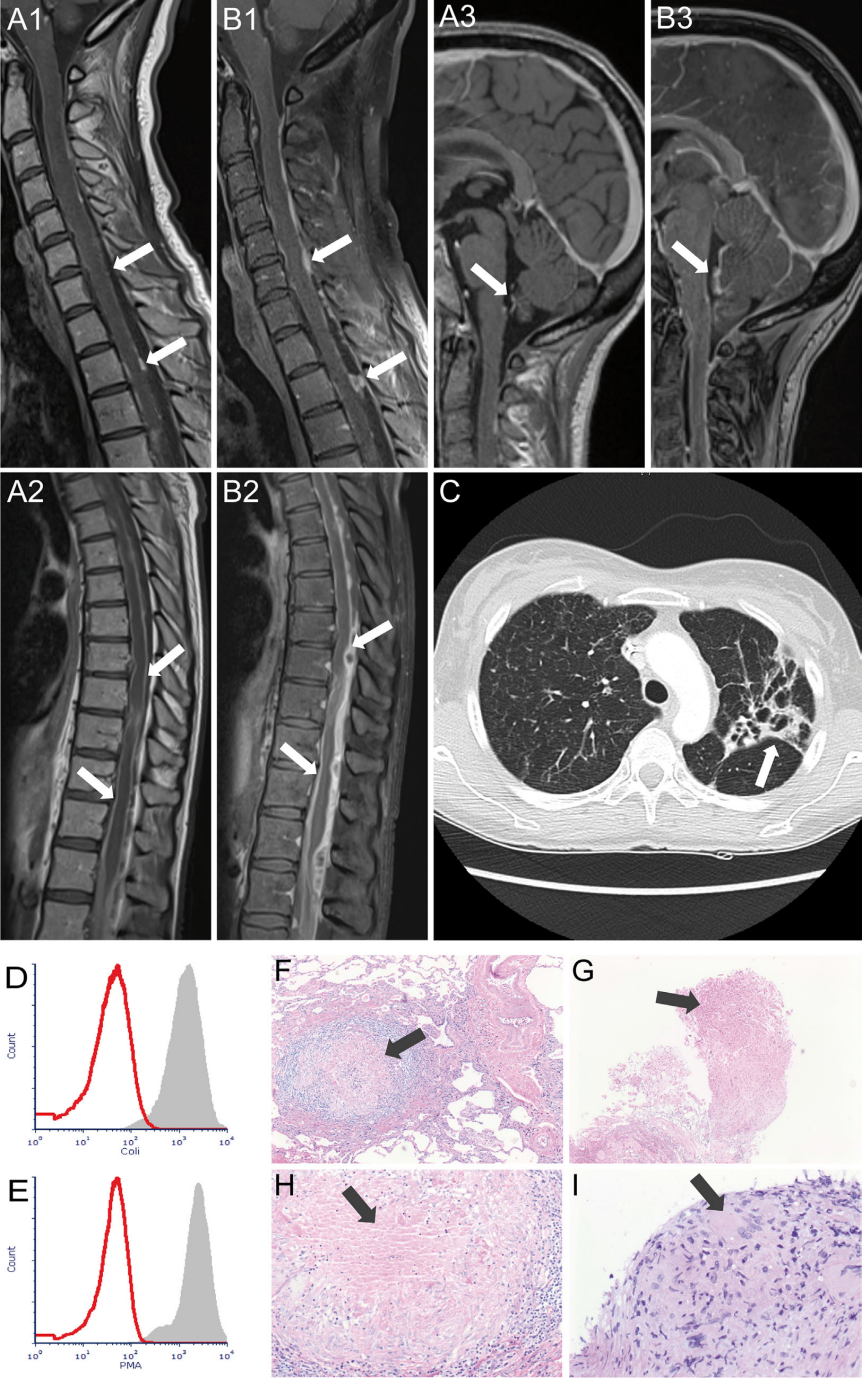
Magnetic resonance imaging demonstrating multiple contrast-enhanced nodular masses along the leptomeninges of cervical (A1), thoracic (A2), and brain (A3) region at admission and progress in all three regions after 3 weeks (B1–B3). Arrows indicate leptomeningeal enhancement with multiple nodular lesions. Chest computed tomography (C) at admission revealed patchy areas of consolidation and cavitation primary in the left upper lung lobe (arrow). (D,E) show the respiratory burst capability of neutrophils. 100 µl of heparinized whole blood of the patient (red histograms) and a healthy control (gray histograms) was stimulated with *Escherichia coli*
